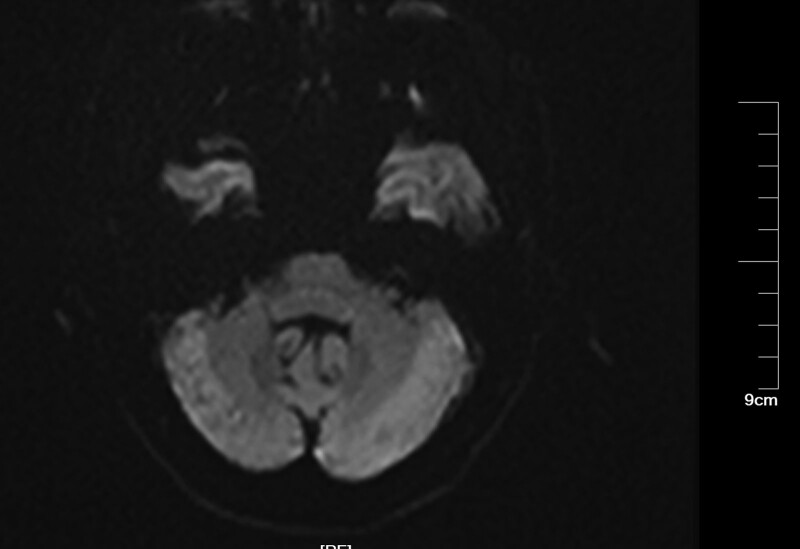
Follow-up imaging 3 months later showed normal brain images on both diffusion-weighted imaging (DWI) and apparent diffusion coefficient (ADC) scans.
